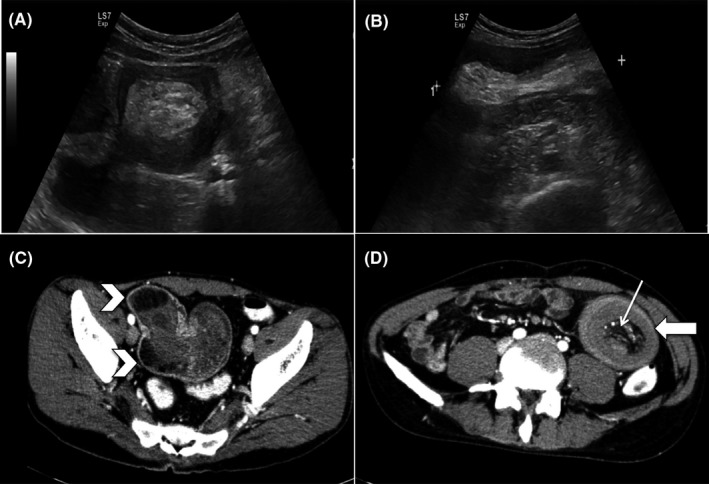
Selected ultrasound (A & B) and CT (C & D) images demonstrating an entero‐enteric intussusception. The ultrasound images reveal characteristic features of “target” sign on transverse view (A) and “pseudokidney” sign (B) on longitudinal view. The CT scan demonstrates “target”‐shaped soft tissue mass with layering effect (white thick arrow). The characteristic intraluminal mesenteric vessels (thin white arrow) are evident. Note is made of a few well‐defined lesions of fat density (−50 to −30 HU) representing lipomas at the most proximal part of the intussusceptum acting as the lead point
